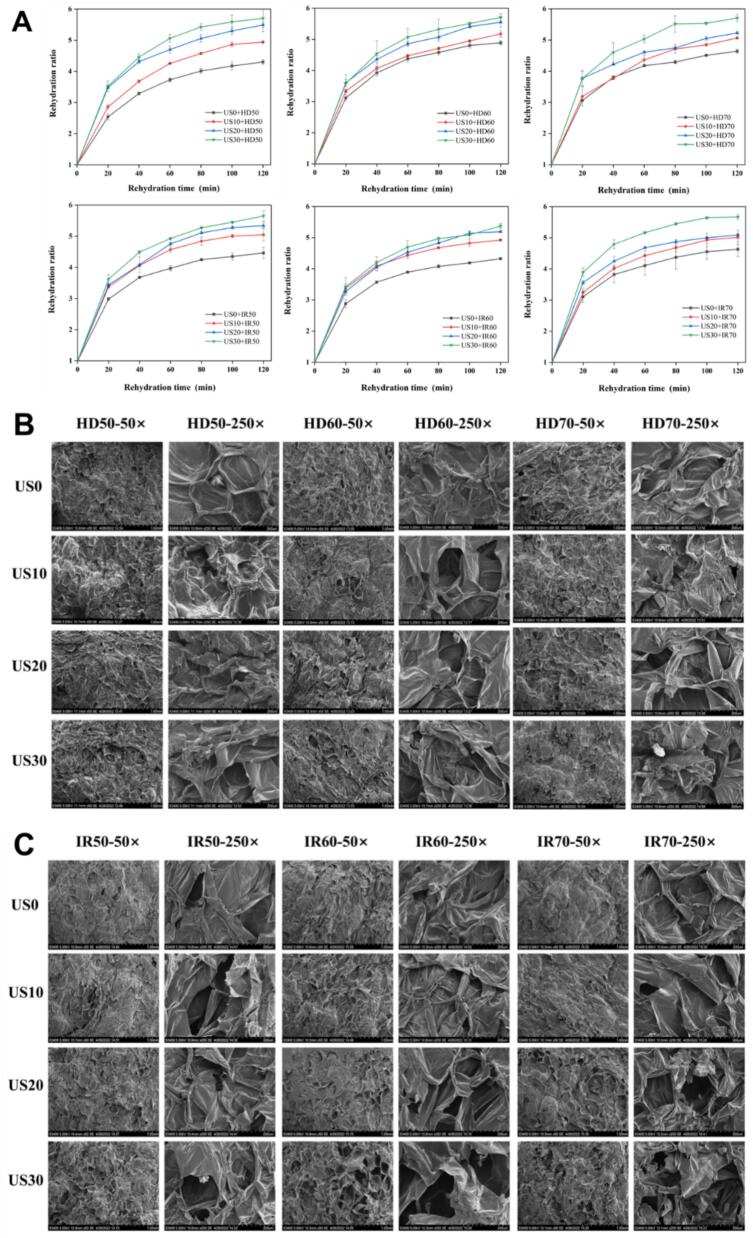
Effects of different ultrasonic pretreatments on rehydration dynamics curve and microstructure of HD apple slices and IR apple slices.
